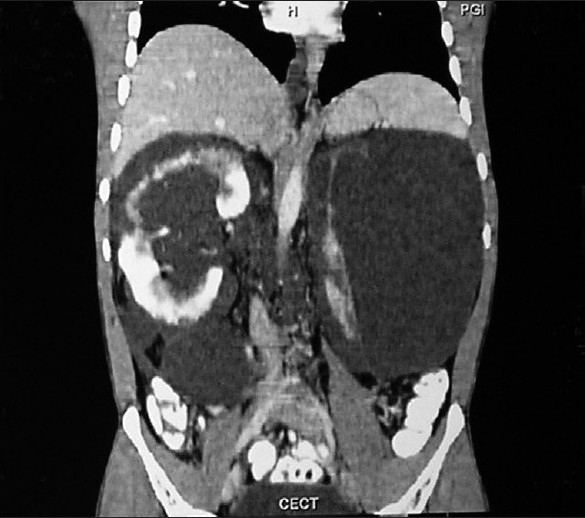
Reformatted contrast enhanced coronal CT image reveals non enhancing extra-parenchymal hypo dense cysts in the perirenal region and the region of the pelvis of both the kidneys (more on the left side) causing distortion of the underlying parenchyma without involving it
